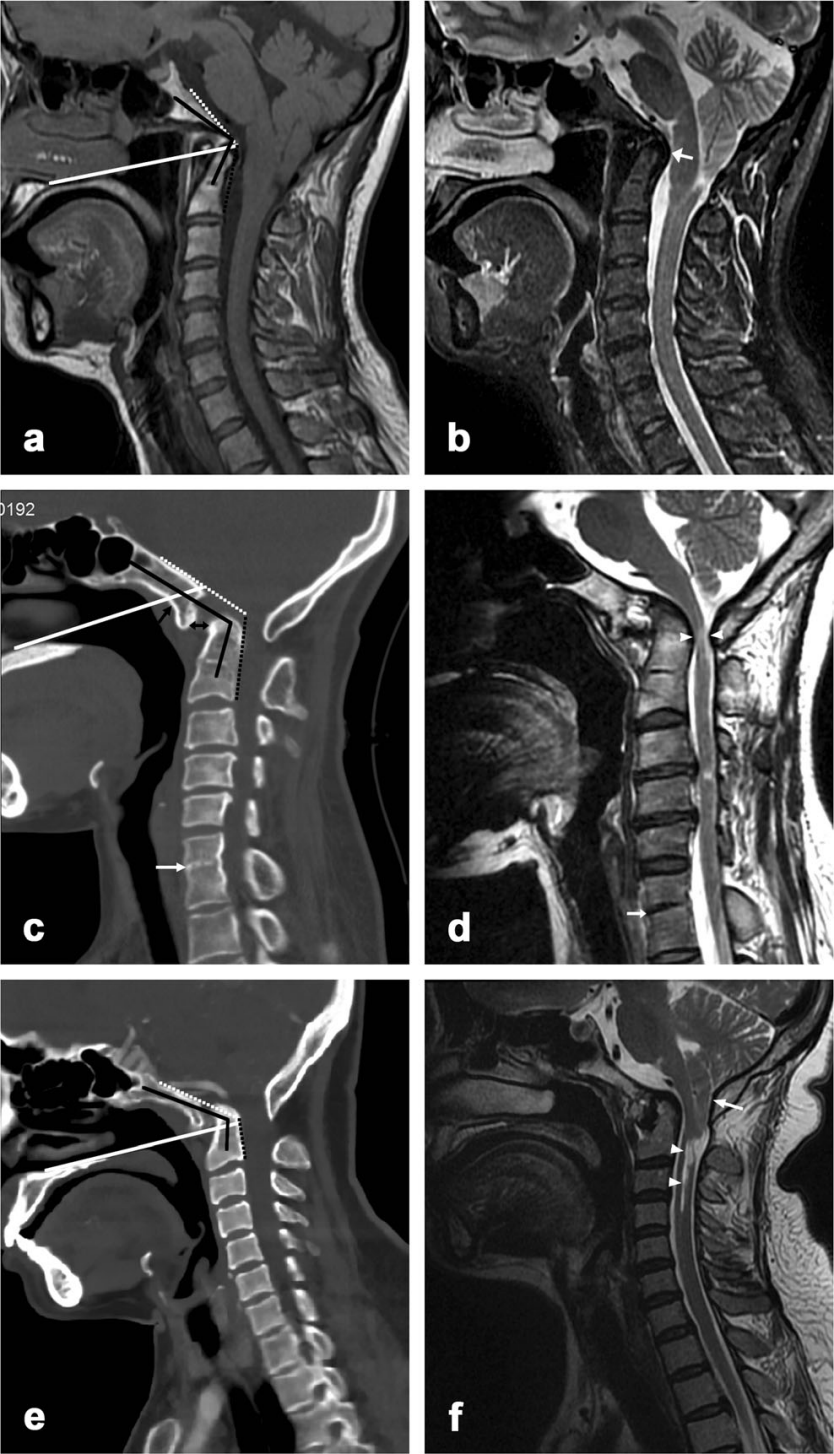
Three typical cases of basilar invagination. Midsagittal T1-weighted (a) and T2-weighted (b) images from a 45-year-old female demonstrating a significantly reduced clivoaxial angle (CXA; intersection of dotted white and black lines, 125°), clivodens angle (CDA; intersection of solid black lines, 100°), and clivopalate angle (CPA; intersection of the dotted white line and the solid white line, 52°), and a Chamberlain line (CL) value of 6 mm. Chiari II malformation and marked medullar compression (arrow) are also shown. Midsagittal CT (c) and T2-weighted (d) images from a 37-year-old female demonstrating abnormal CXA (118°), CDA (111°), and CPA (49°), and a CL value of about 6 mm. Atlas occipitalization (comma-shaped configuration, black arrow), atlantoaxial subluxation (double arrow), C6–7 assimilation (white arrow), as well as foramen magnum stenosis, cervical cord compression, and degeneration (white arrowhead) are also shown. Midsagittal CT (e) and T2-weighted (f) images from a 48-year-old female demonstrating abnormal CXA (122°), CDA (111°), and CPA (45°), and a CL value of 10 mm. Note the atlas occipitalization, atlantoaxial subluxation, syringomyelia (arrowhead), and Chiari I malformation (arrow)
